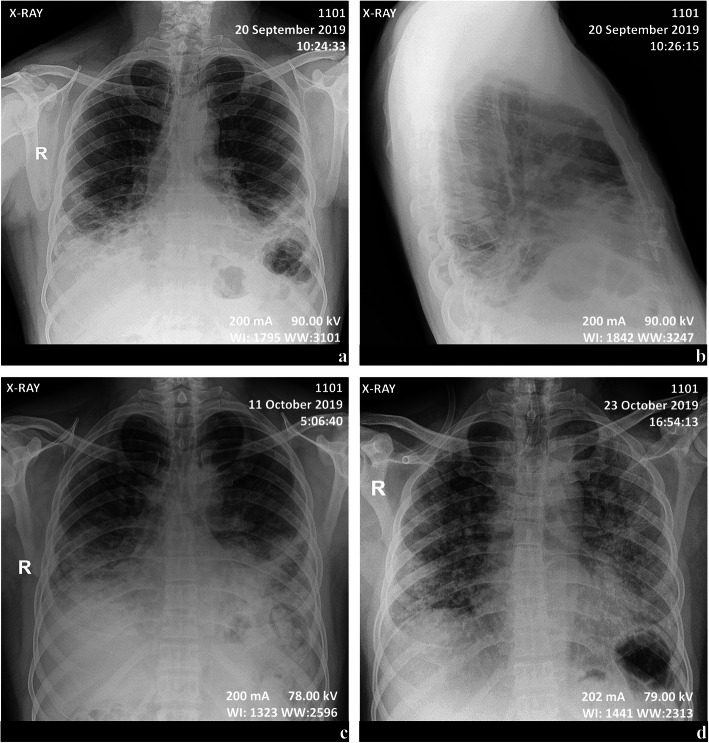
Serial chest X-rays of Patient 1. Multifocal then diffuse airspace disease in a 55-years-old man. Bibasal lung consolidation with assorted trabeculae, especially in the posterior regions, overhung by discreet hazy opacities (a, b). Follow-up showing a cranially progress of the predominant inhomogeneous consolidation still coexisting with a clearly diffuse ground-glass (c). There is apparent replacement of alveolar densification by diffuse reticular interstitial marks with some few parahilar nodules (d)
